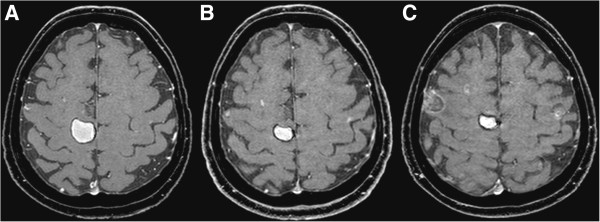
Gadolinium-enhanced T1-weighted magnetic resonance images of cerebral metastases. A. MRI of the brain before treatment with vemurafenib. B. MRI at presentation with visual loss. C. MRI four weeks after cessation of treatment.
